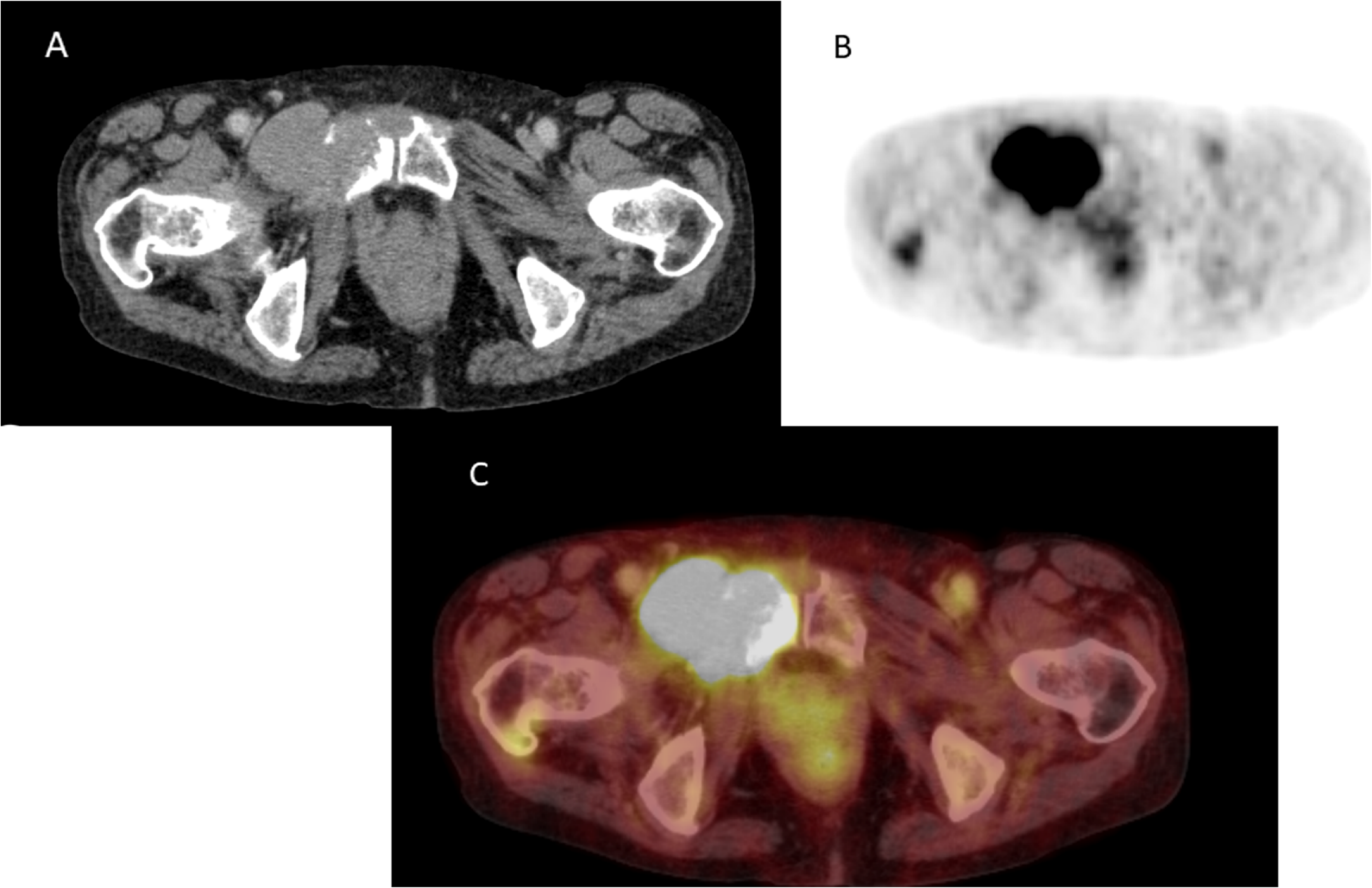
Myeloid sarcoma of the pelvis in a female patient with known acute myeloid leukemia. a Axial contrast media enhanced computed tomography shows a homogenous bone lesion of the right pubic bone with infiltration of the adjacent muscle. b On F18-fluorodexyglucose-Positron-emission tomography a high tracer uptake can be appreciated of the lesion. c Corresponding fused F18-fluorodexyglucose-Positron-emission tomography
